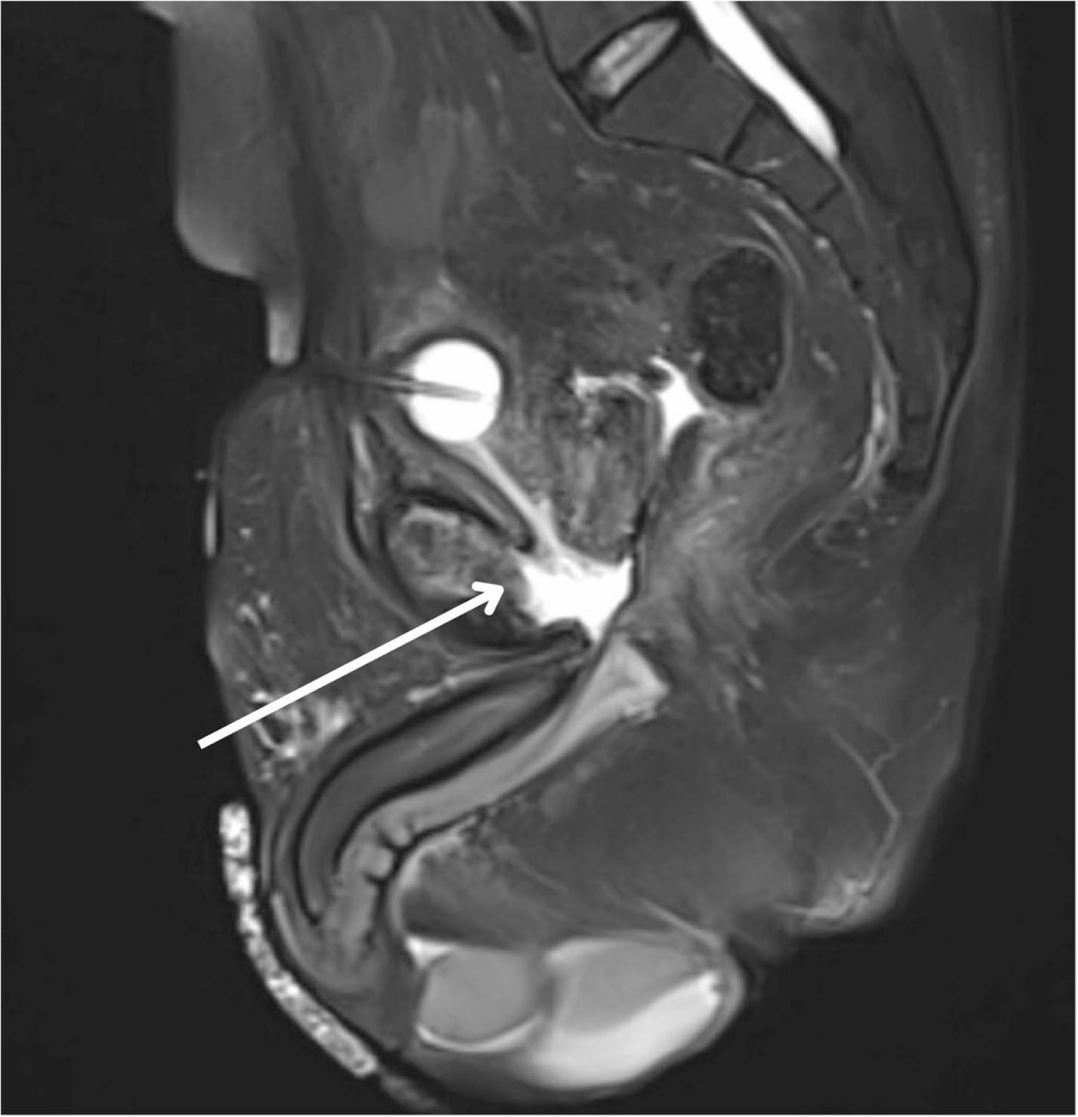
Sagittal view of pelvic MRI demonstrating fistulous tract between urethra and pubic symphysis
